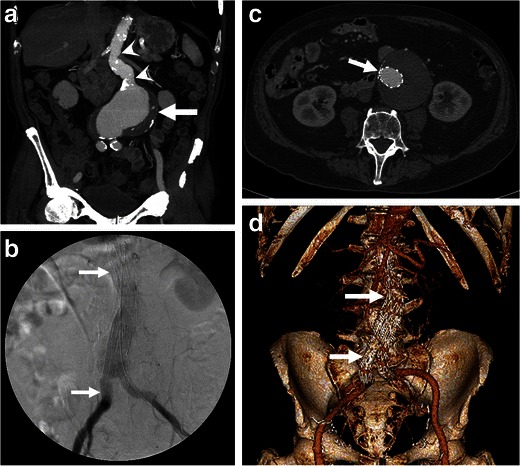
Endovascular aneurysm repair. a Coronal enhanced CT image of an 85-year-old man presenting with an impending AAA rupture (same as Fig. 5) demonstrates a large AAA (*white arrow*) with a long proximal neck (*white arrowheads*). b Fluoroscopy image, c axial, and d 3D rendering of the same patient show a deployed aorto-biiliac stent-graft (*white arrows)*
